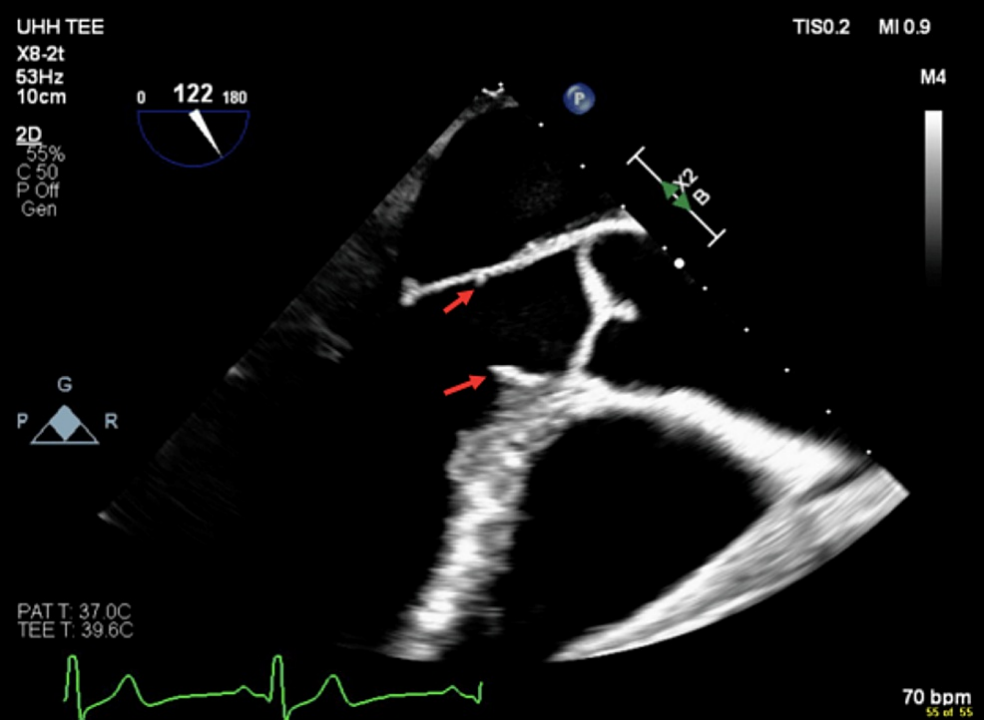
Transesophageal echocardiogram demonstrating the presence of a subaortic membrane.
